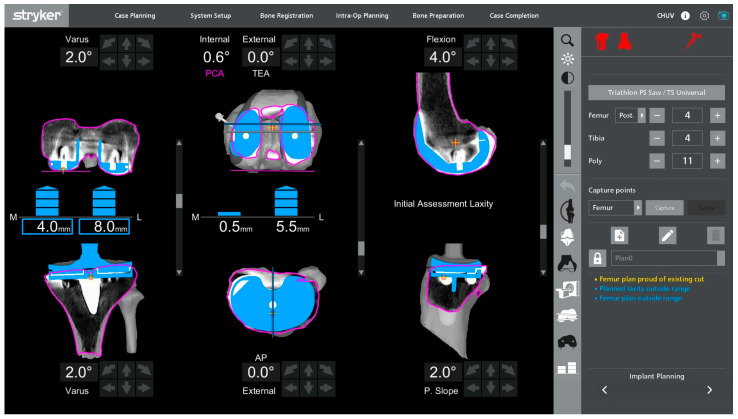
Screenshot of the Mako software showing the functional alignment plan with adjusted femoral and tibial resection levels on the three-dimensional CT-based reconstruction.
